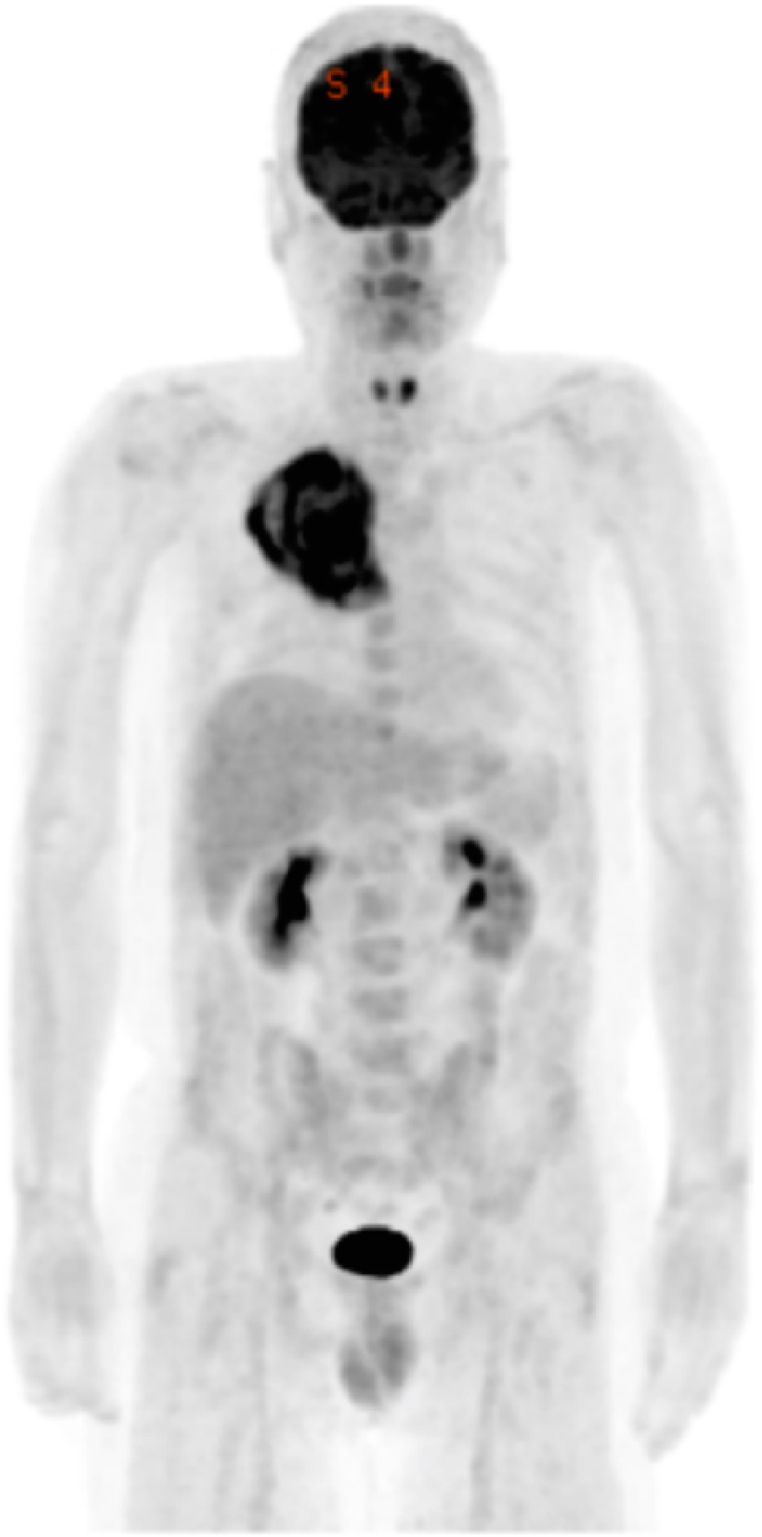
Positron emission tomography (PET) with 18F-fluorodeoxyglucose (FDG) shows the accumulation of the tracer in a tumor in the right upper lobe.
